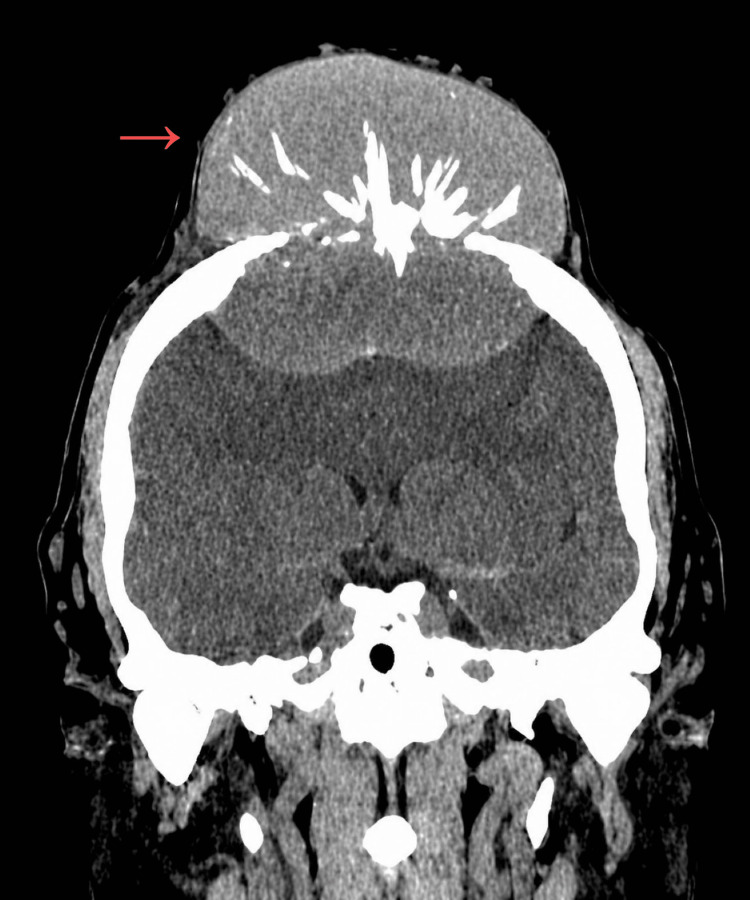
Coronal CT image showing a giant osteolytic calvarial lesion Contrast-enhanced coronal CT image demonstrating a large expansile osteolytic lesion centered at the cranial vertex, with internal bony spicules and a soft-tissue component producing local mass effect on the adjacent brain parenchyma. CT: computed tomography
